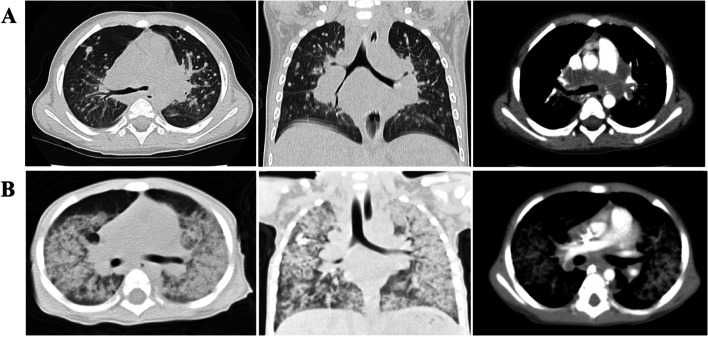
Lung windows from high-resolution CT scan of the patients. A Diffused nodules on two lungs with enlarged hilar lymphatic nodules (white arrows) in P1; B Bilateral distributing ground-glass opacities in P3
